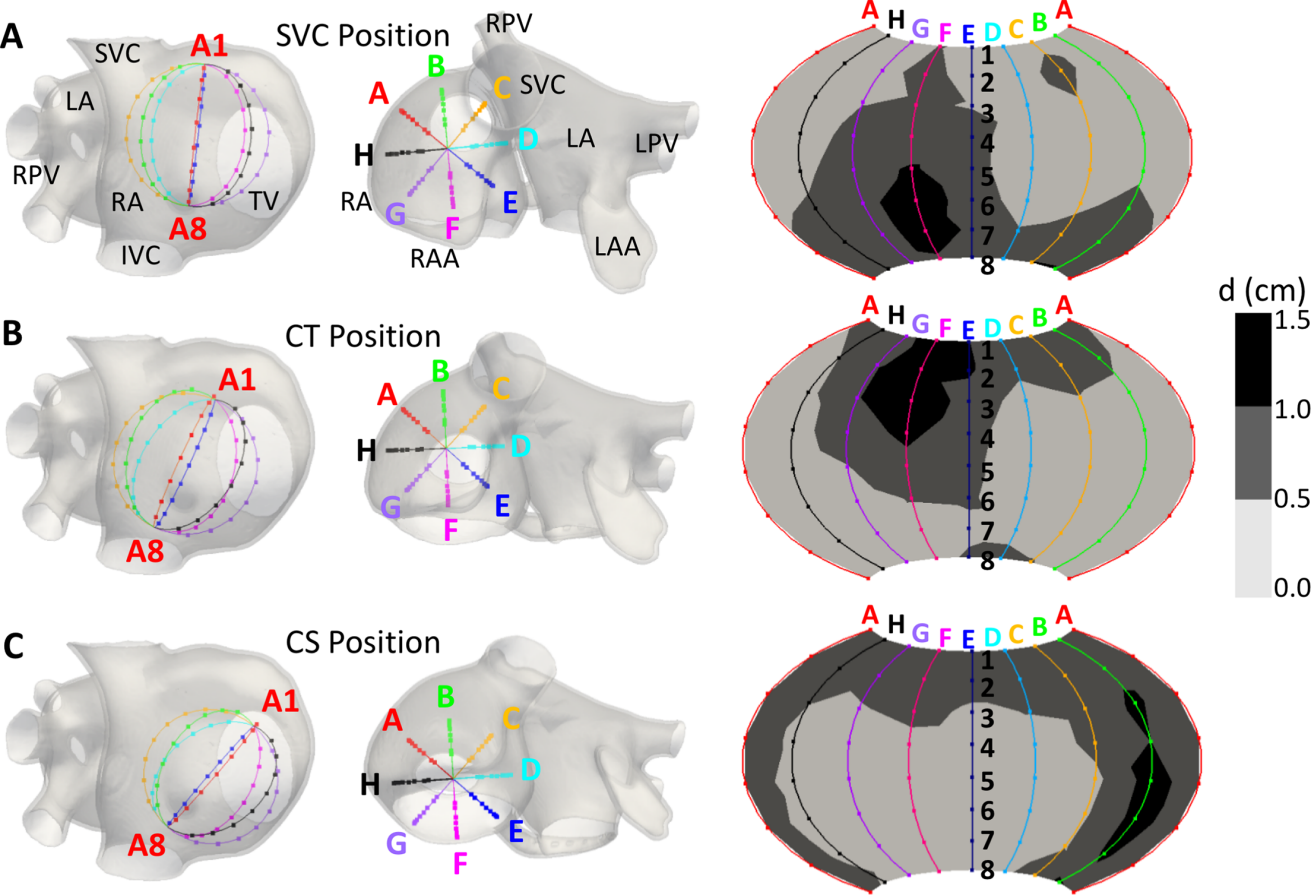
Positions of the 64-pole basket mapping catheter in the RA. A-C) SVC, CT and CS position (first and second column) and electrode-endocardium distance maps for the 64 electrodes in each position (third column). SVC and IVC: superior and inferior vena cava; LA and RA: left and right atrium; LPV and RPV: left and right pulmonary veins; TV: tricuspid valve; LAA and RAA: left and right atrial appendage.
